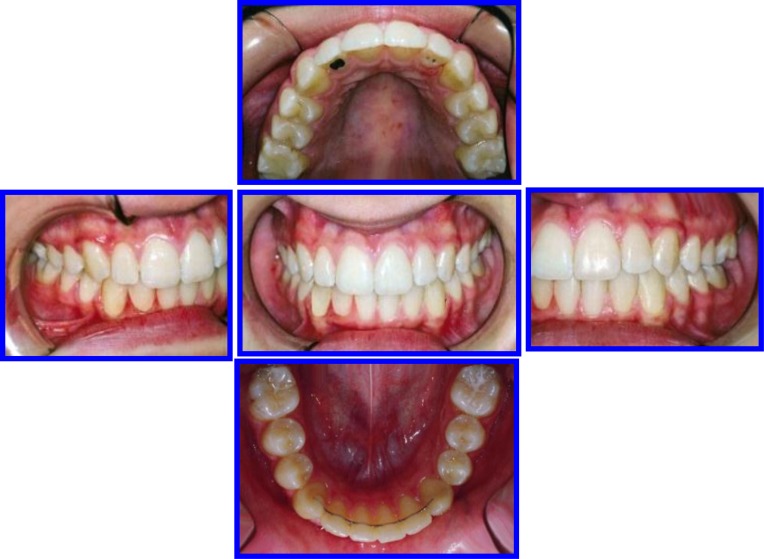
End of Phase II treatment showing radiographs, facial & occlusal features. Case #2.
